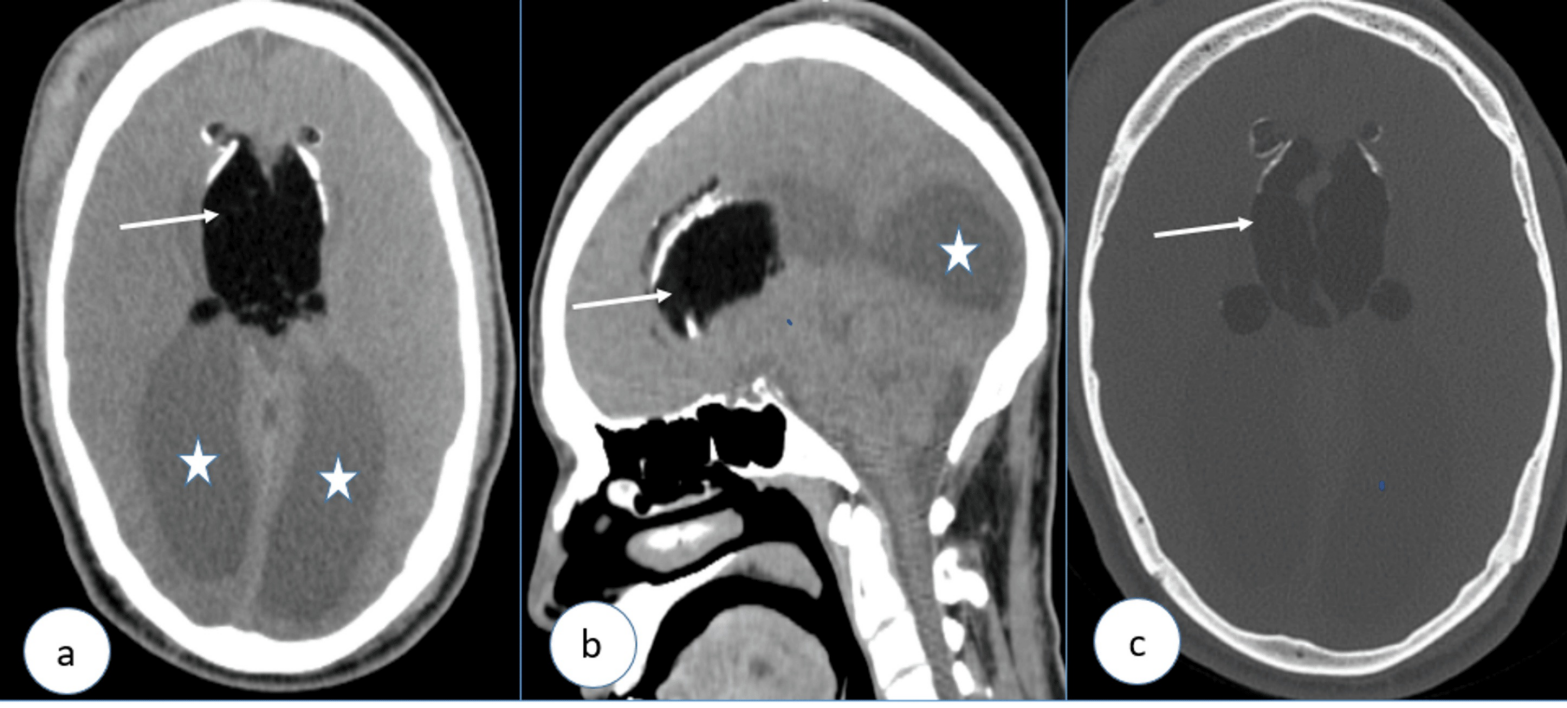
Brain CT in parenchymal window with axial (a) and sagittal (b) views, and in bone window (c), showing an intra-axial fatty lesion with peripheral calcifications (white arrows) located in the interhemispheric fissure and centered on the corpus callosum. The ventricles are widely spaced with colpocephaly (stars) in keeping with corpus callosal dysgenesis CT: computed tomography
